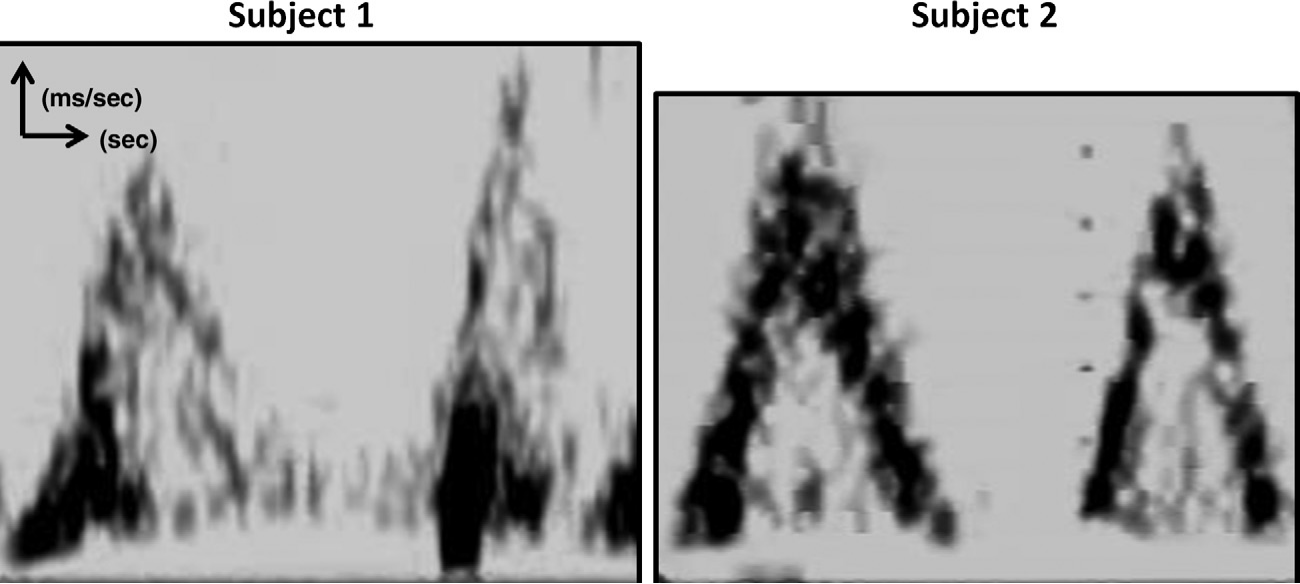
Comparing the ability of VFT_kinematic_ and VFT_standard_ to differentiate between two similar Doppler *E*-waves. The *E*-wave image on the left (marked Subject 1) is from Subject 5 (Table 3; normal group). The image on the right (marked Subject 2) is from Subject 17 (PN group). VFT_standard_ can not differentiate between these two waves while VFT_kinematic_ can differentiate between them. See text and Table 4 for details. VFT, vortex formation time.
